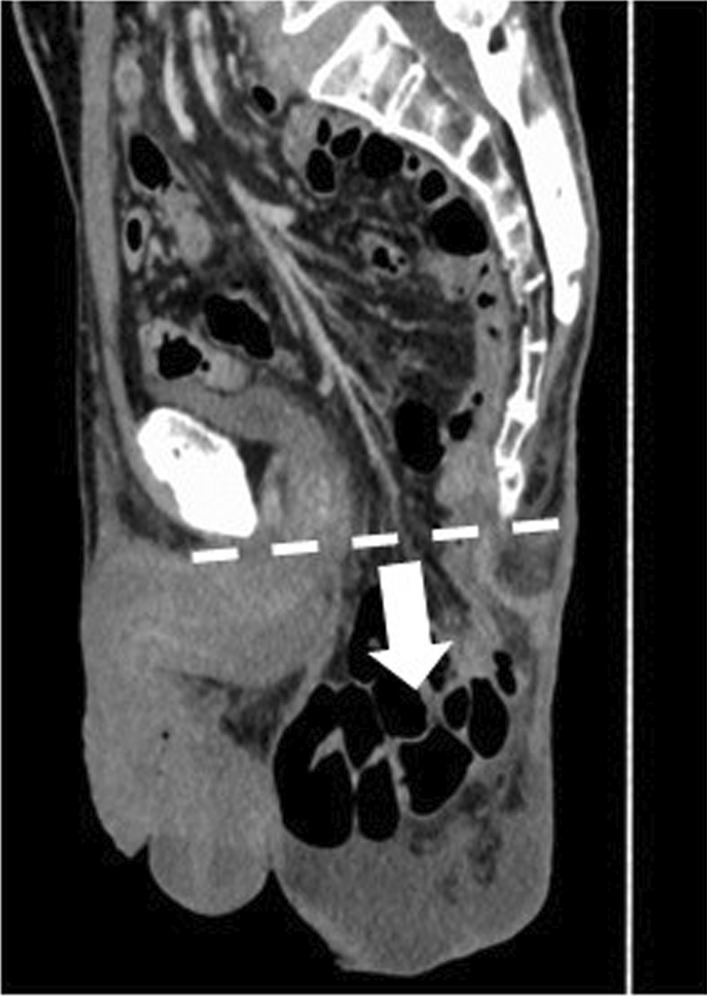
Diagnosis of postoperative perineal hernia by computed tomography is defined as the downward displacement of the intestine beyond the line described by computed tomography from the inferior margin of the pubis to the end of the coccyx
